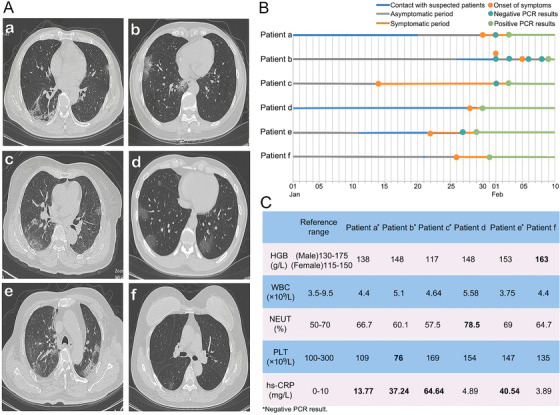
The characteristics of six patients admitted to hospital. (A) The chest CT images showed ground‐glass opacity (GGO) lesions in both lungs; (B) the asymptomatic period and RT‐PCR test results; and (C) the laboratory examinations results
